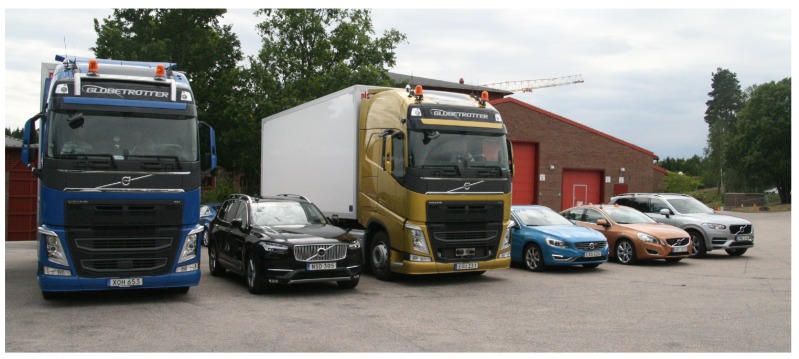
The six vehicles used during the measurements, from the left: Truck Blue, XC90 Black (large SUV), Truck Gold, S60 (mid size sedan), V60 (mid size wagon), and XC90 Silver.
